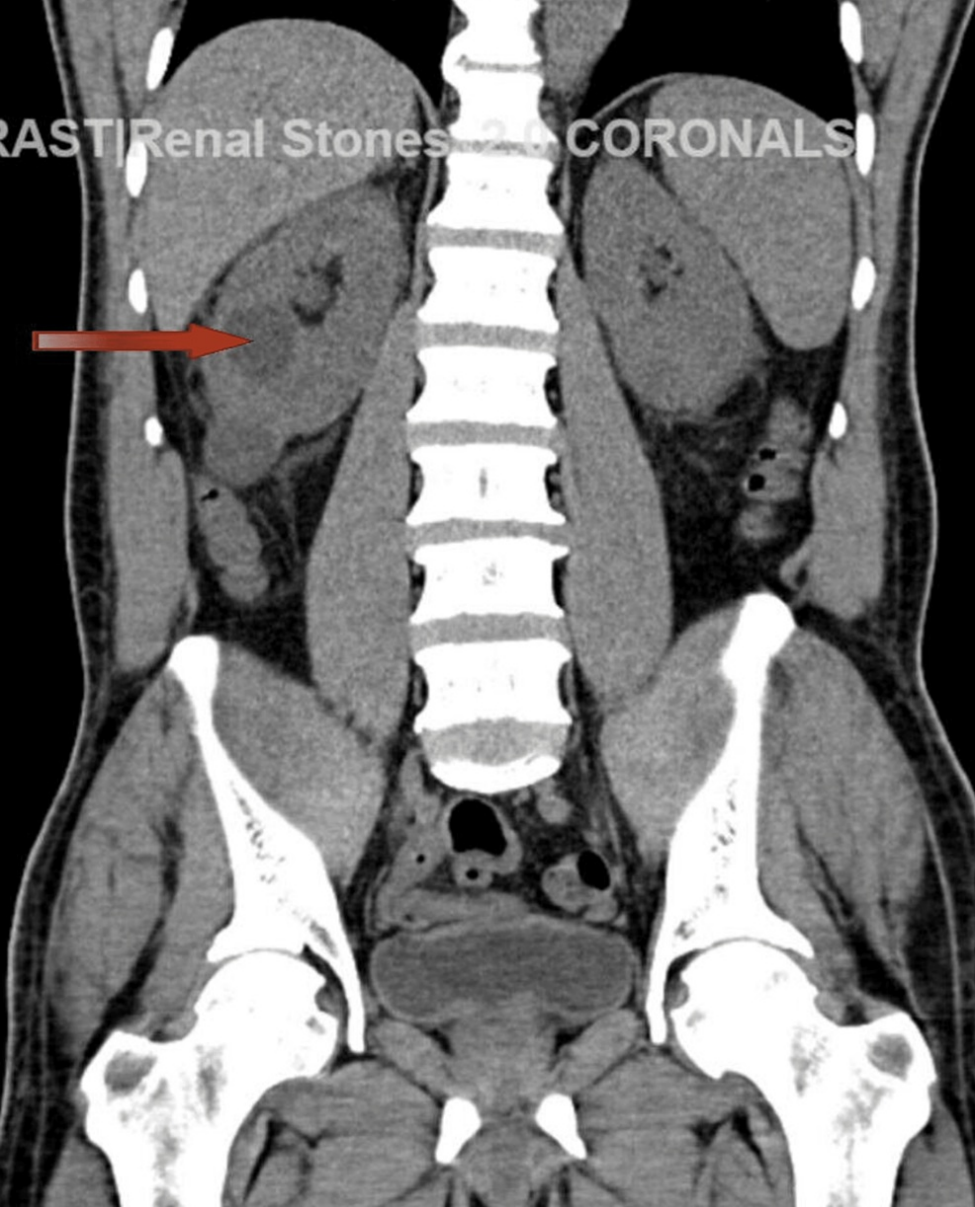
Computed tomography (CT) of the abdomen and pelvis in coronal section shows renal cyst on the right side (red arrow)
